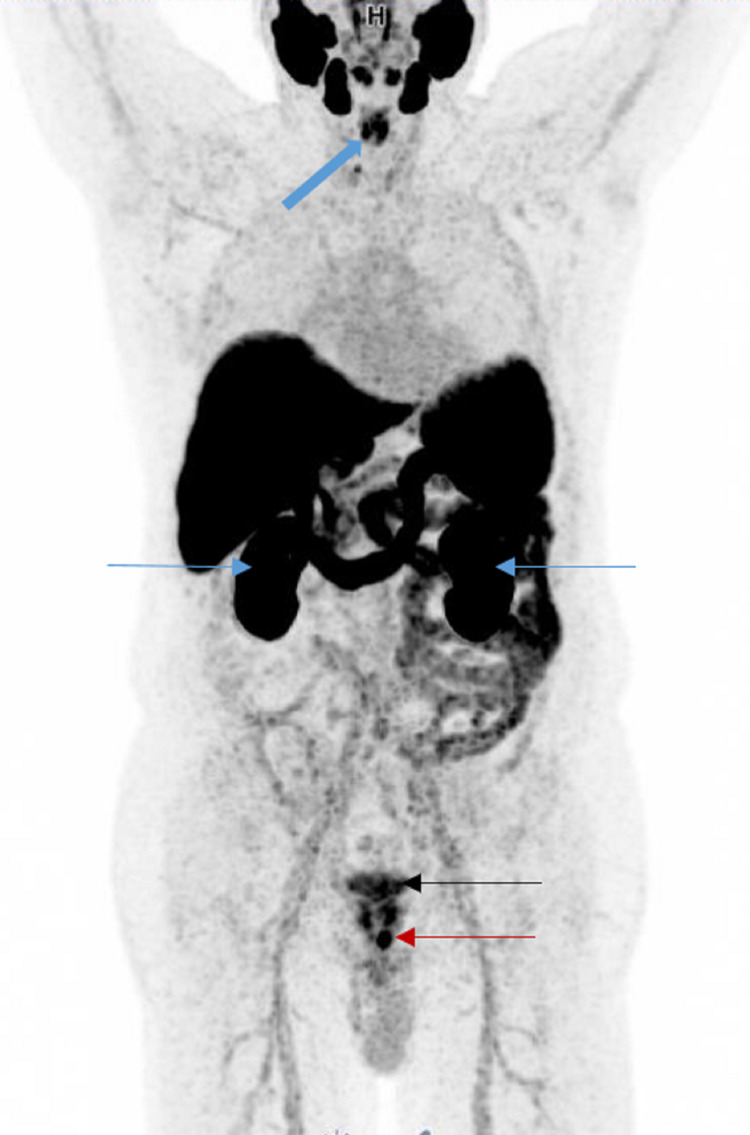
Normal biodistribution 68Ga PSMA MIP image shows physiological activity in the kidneys (blue thin arrows) and urinary bladder (black arrow) from excretion. Note also the physiological activity in the oropharyngeal mucosa (blue thick arrow). Local tumor recurrence is seen in the prostatic bed (red arrow).
